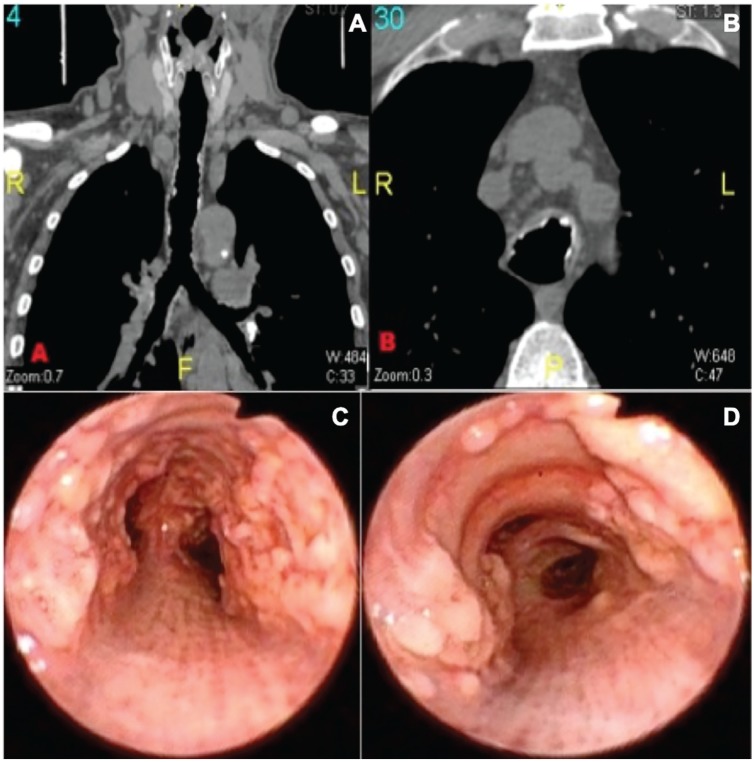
(A) Thoracic coronal computed tomography (CT) with a mild beaded appearance of the trachea with no airway stenosis. (B) Thoracic axial CT scan showing mild thickening of the anterolateral walls of the trachea with nodules, which are calcified. (C and D) Bronchoscopy showing diffuse submucosal nodules with an intact overlying mucosa, compromising the anterolateral walls and extending to the main bronchi. Observe the intact membranous trachea.
